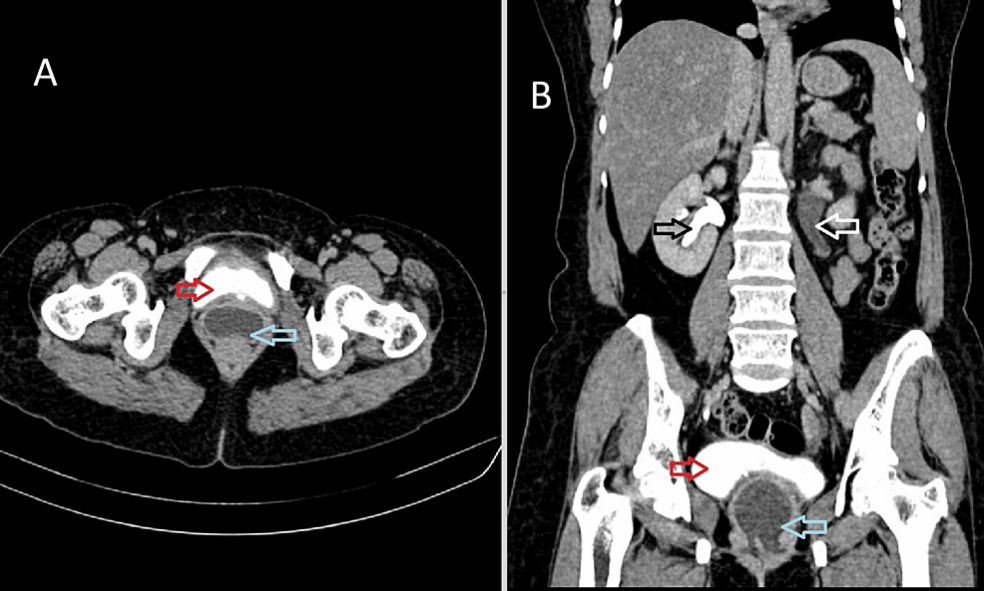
Axial (A) and coronal (B) CT urography excretory phase (3 mins), showing normal excretion of contrast in the right renal collecting system (black arrow) filling the bladder (red arrow), no excretion of contrast in the left renal collecting system (white arrow). The cavity in the vaginal stump appears distended with fluid-filled collection within, possibly urine (blue arrow). CT: Computed tomography
